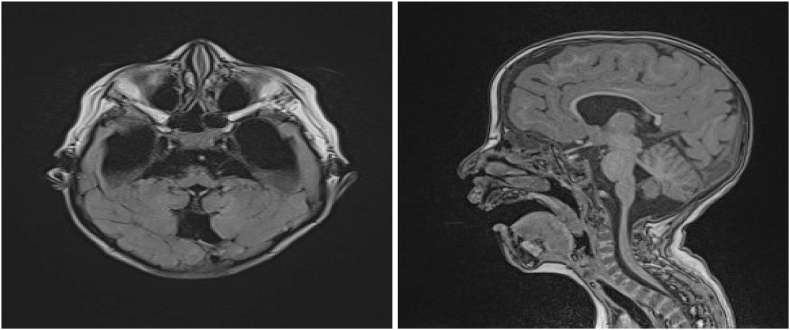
Brain MRI with axial FLAIR and sagittal T1 sequence showing partial hypoplasia of the inferior vermis with communication of the 4th ventricle and the large cistern and ventricular dilatation without other associated malformations.
